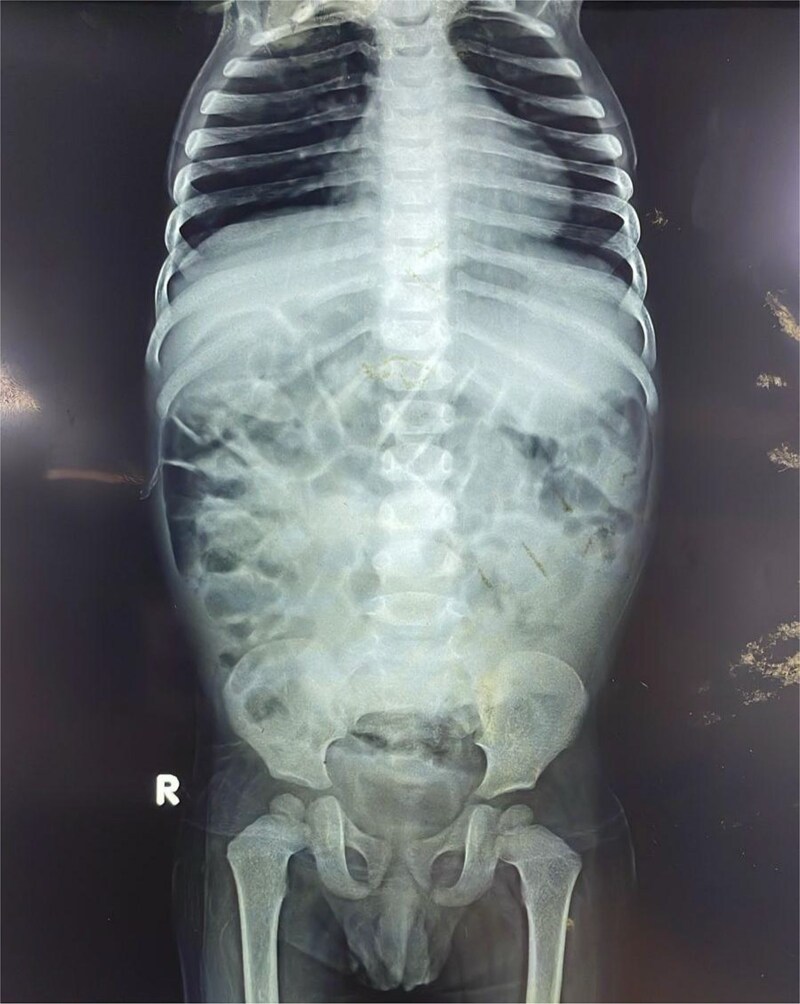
Anteroposterior abdominal radiograph demonstrating diffusely air-distended small bowel loops consistent with a partial small bowel obstruction. No significant air-fluid levels or evidence of pneumoperitoneum is visible.
